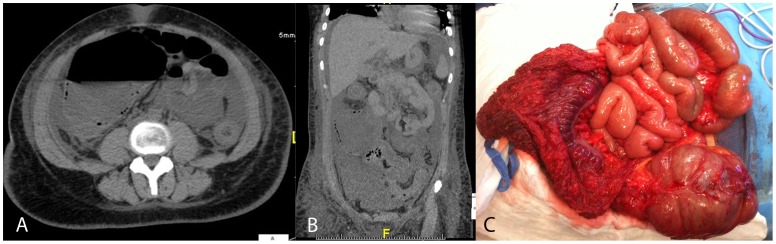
Patient with EHEC O104, Noro-virus, and Clostridium difficile co-infection. CT scan showing massive swelling of the intestinal wall, extreme dilation of the right colon with intramural air (a and b). Intraoperative situs with extreme wall thickening of the ascending colon and incipient perforation of the cecum (c).
